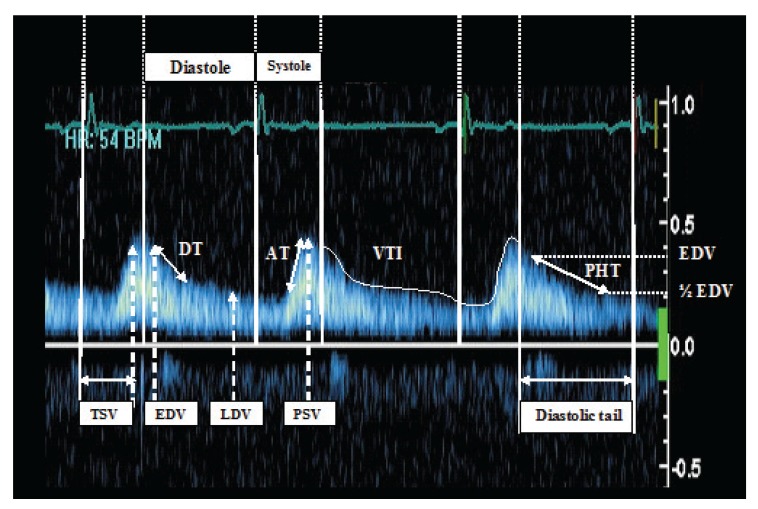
Continuous-wave Doppler echocardiography of the abdominal aorta. AT : systolic acceleration time is measured from the onset of the systolic upstroke to the systolic peak. DT: deceleration time is measured from Peak E velocity to the point where the slope of the slowing flow would intercept the baseline. EDV: early diastolic velocity: maximum diastolic velocity on early diastole. LDV: late diastolic velocity: maximum diastolic velocity on late diastole. PHT: pressure half-time (of diastole) is the time interval for the peak diastolic pressure gradient to be reduced by one half. PSV: peak systolic velocity: maximum systolic velocity. TSV: time to peak systolic velocity: time beginning from onset of QRS complex to peak systolic velocity. VTI: velocity time integral: the area under the curve, shown in both systole and diastole.
